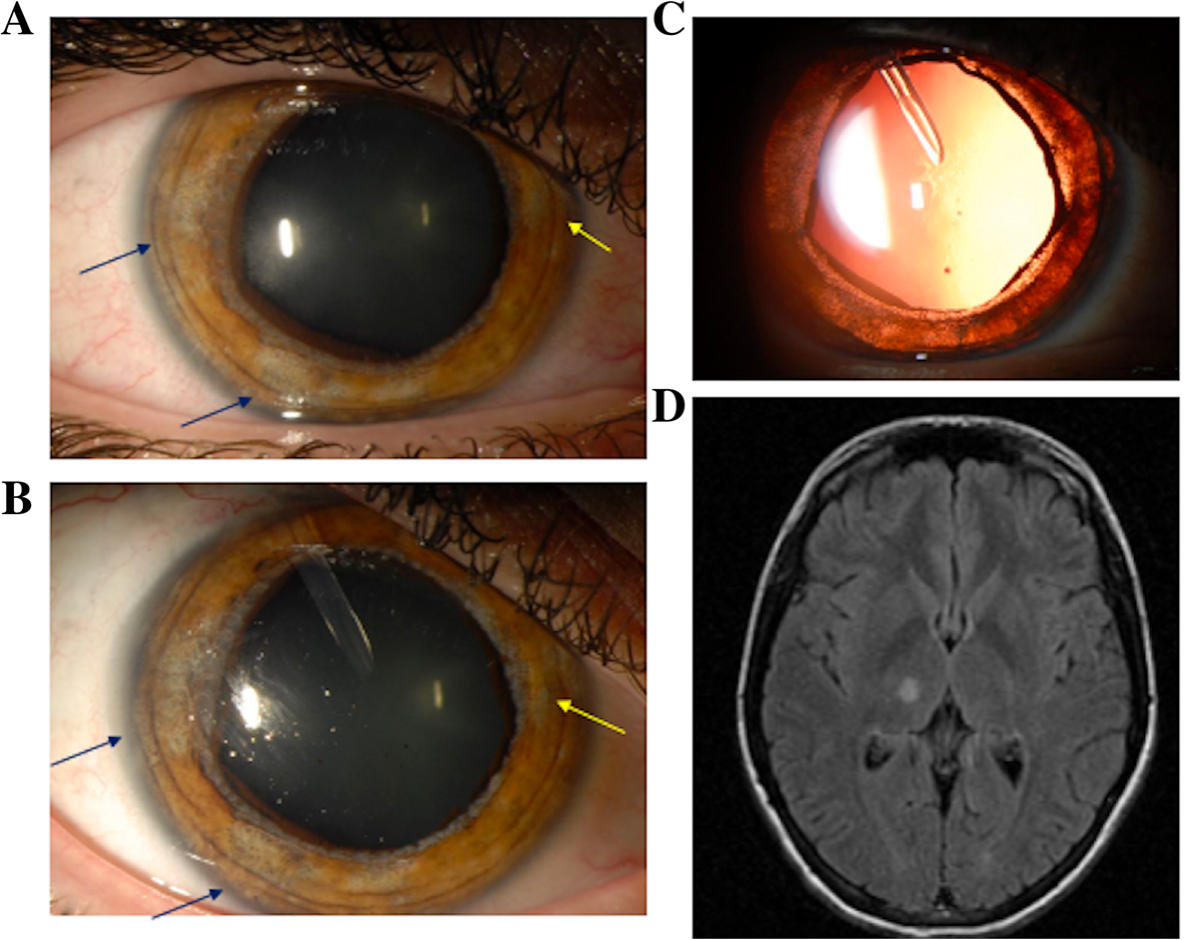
Slit-lamp photo showed multifocal patches of iris atrophy temporally (blue arrows) and nasally (yellow arrow) at presentation (a). The areas of atrophy enlarged with increased pigment deposition at follow-up (b). These areas are readily visualized as diffuse transillumination defects with retroillumination (i.e. orange/red reflex off the retinal surface) which is visualized through the atrophic iris stroma and epithelium (c). A T2 FLAIR magnetic resonance image after the patient’s stroke showed lacunar infarcts within the right thalamus (d)
